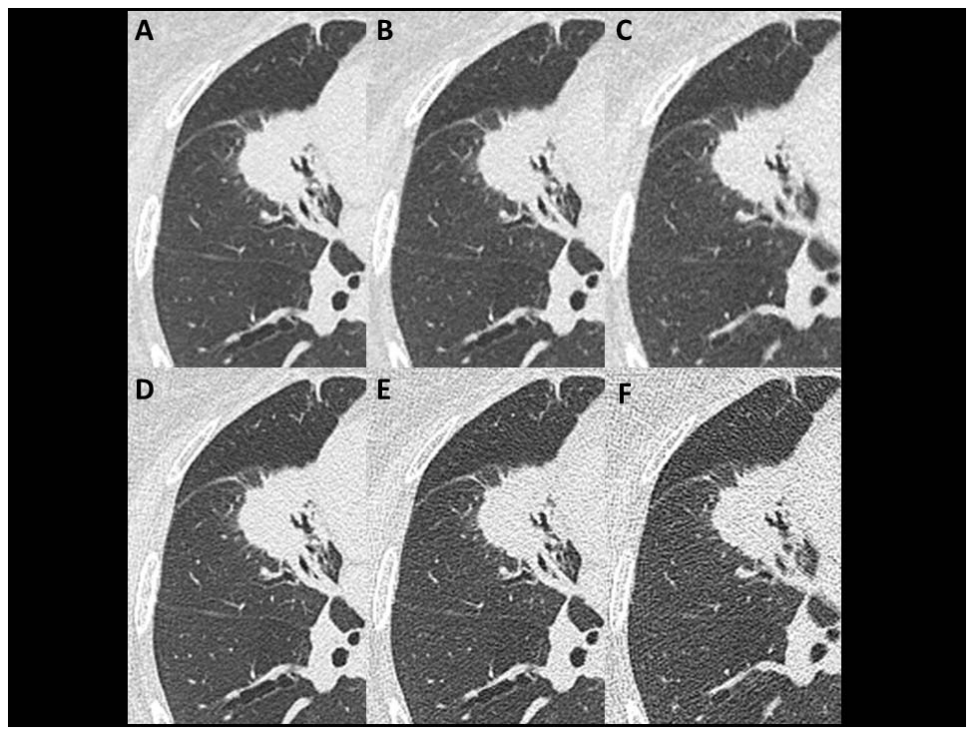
Axial plain chest CT images with a solid lung mass in the right middle lobe (75-year-old female weighing 56 kg). Images are arranged as in Figures 1 and 2. Spiculae were found on all images, while density heterogeneity inside the mass was severe on images at 60 mA without AIDR3D (F).
